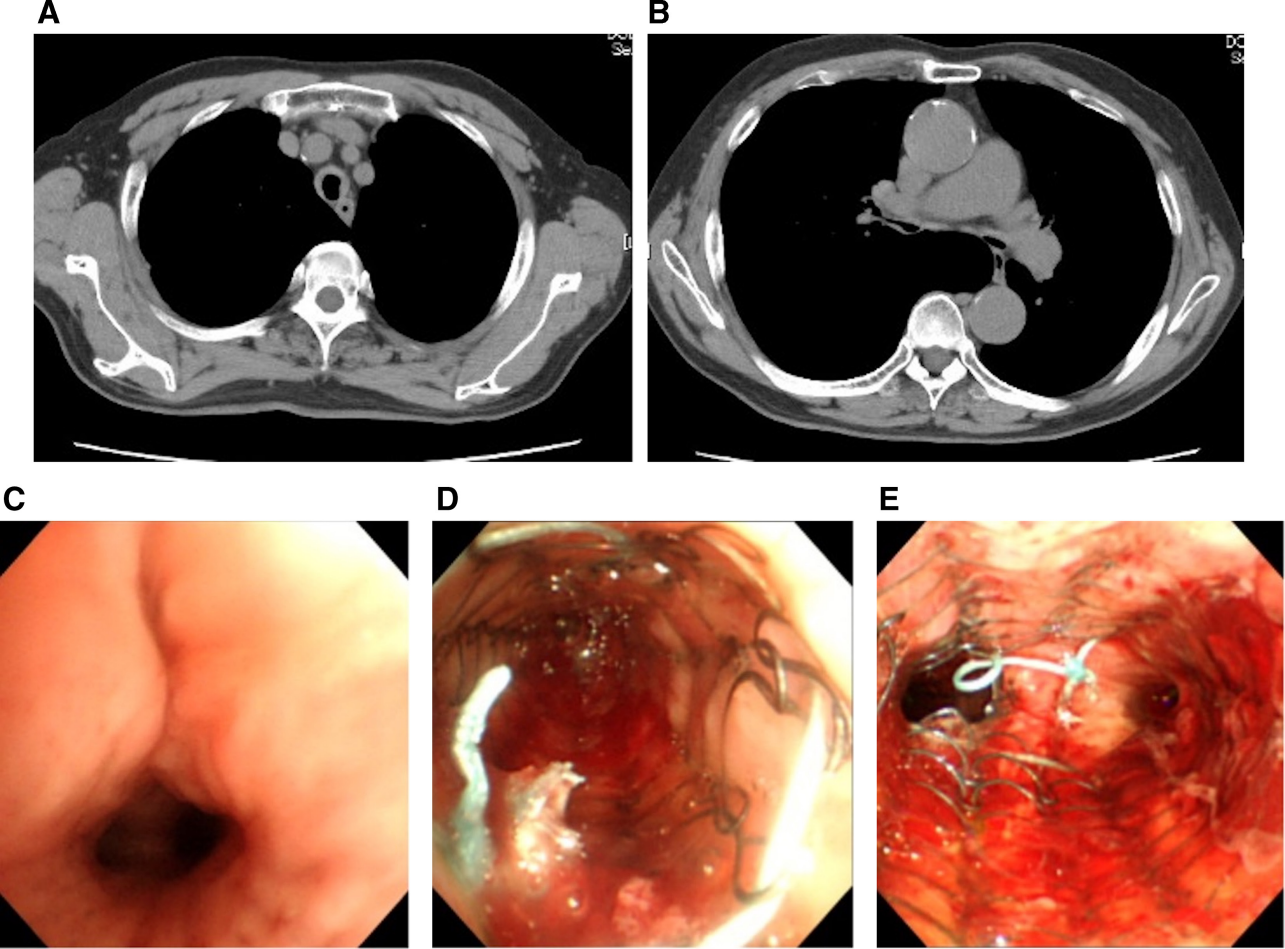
(A, B) Chest CT revealed diffuse bronchial wall thickening. (C–E) An uncovered Ultraflex SEMS (14 × 40 mm) was deployed in the right bronchus intermedius and another uncovered Ultraflex SEMS (20 × 60 mm) was placed in the trachea.
